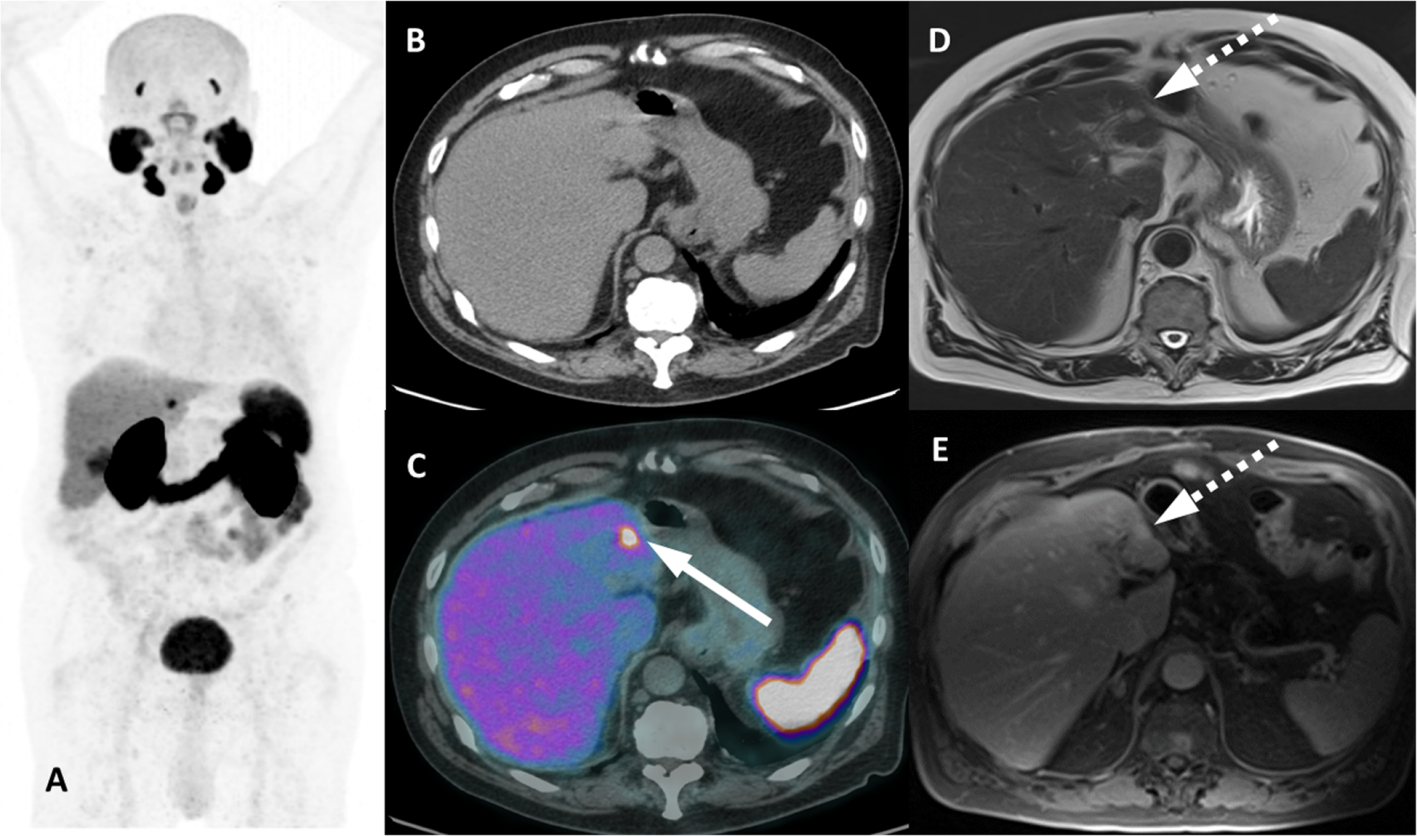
Benign hepatic hemangioma with PSMA uptake. ^68^Ga-PSMA-PET MIP (a) and axial PET/CT (c) images show an isolated moderate focal uptake on the left liver lobe (arrow) not seen on non-contrast enhanced CT in (b), which was subsequently caractherized as a typical hemangioma (dashed arrows) in axial T2w (d) and axial T1w after gadolinum (e). Contrast-enhanced CT performed 10 years earlier (not shown) had already characterized the lesion
